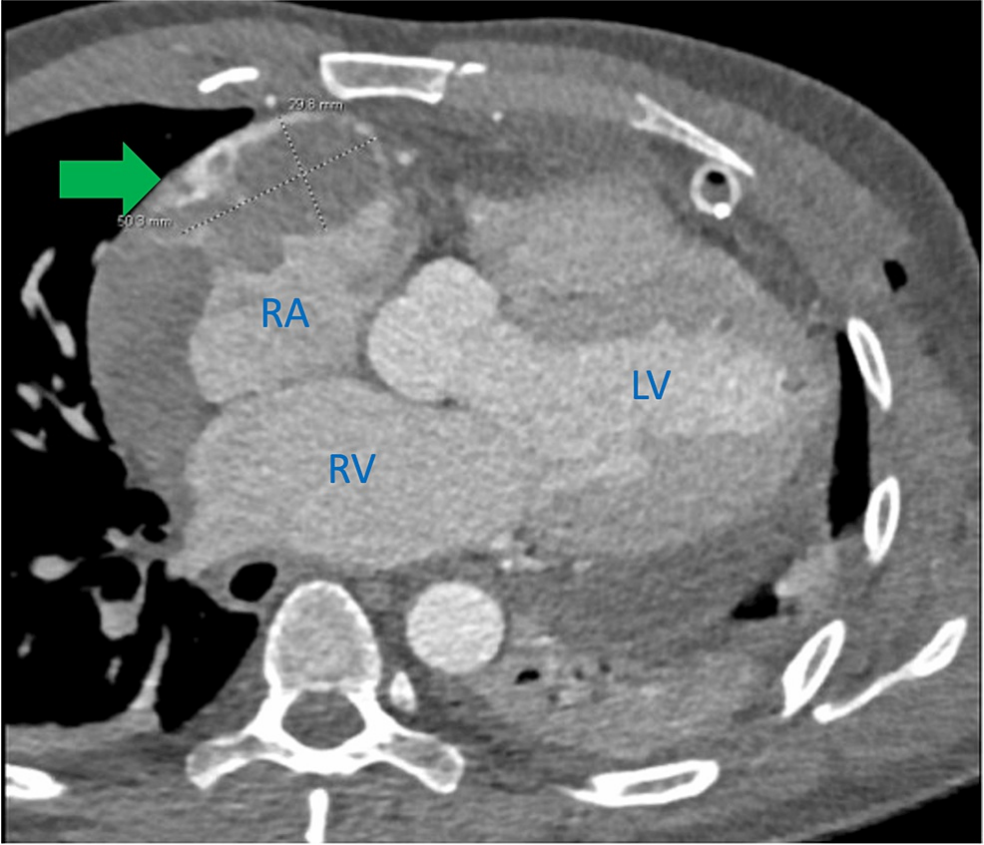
CTA post pericardial window showing an abnormal irregular contour of the right atrial free wall. An adjacent not enhancing hypodense mass (green arrow) measuring 5.0 cm x 2.9 cm surrounded by contrast is also evidenced which is consistent with right atrial rupture contained by a hematoma. CTA: computed tomography angiography Green arrow: hypodense mass in right atrium free wall RA: right atrium RV: right ventricle LV: left ventricle
